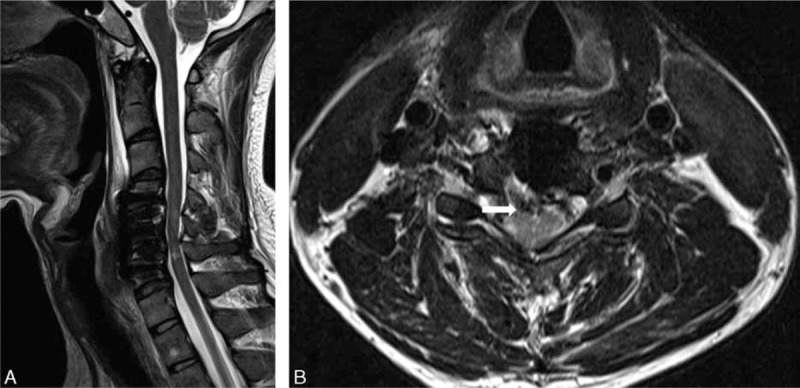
MRI taken 3 weeks postoperatively showed high signal intensity in the spinal cord at C4–C5 and C5–C6 (A), as well as adhesions of the spinal cord to the PLL (arrow) (B). MRI = magnetic resonance imaging, PLL = posterior longitudinal ligament.
